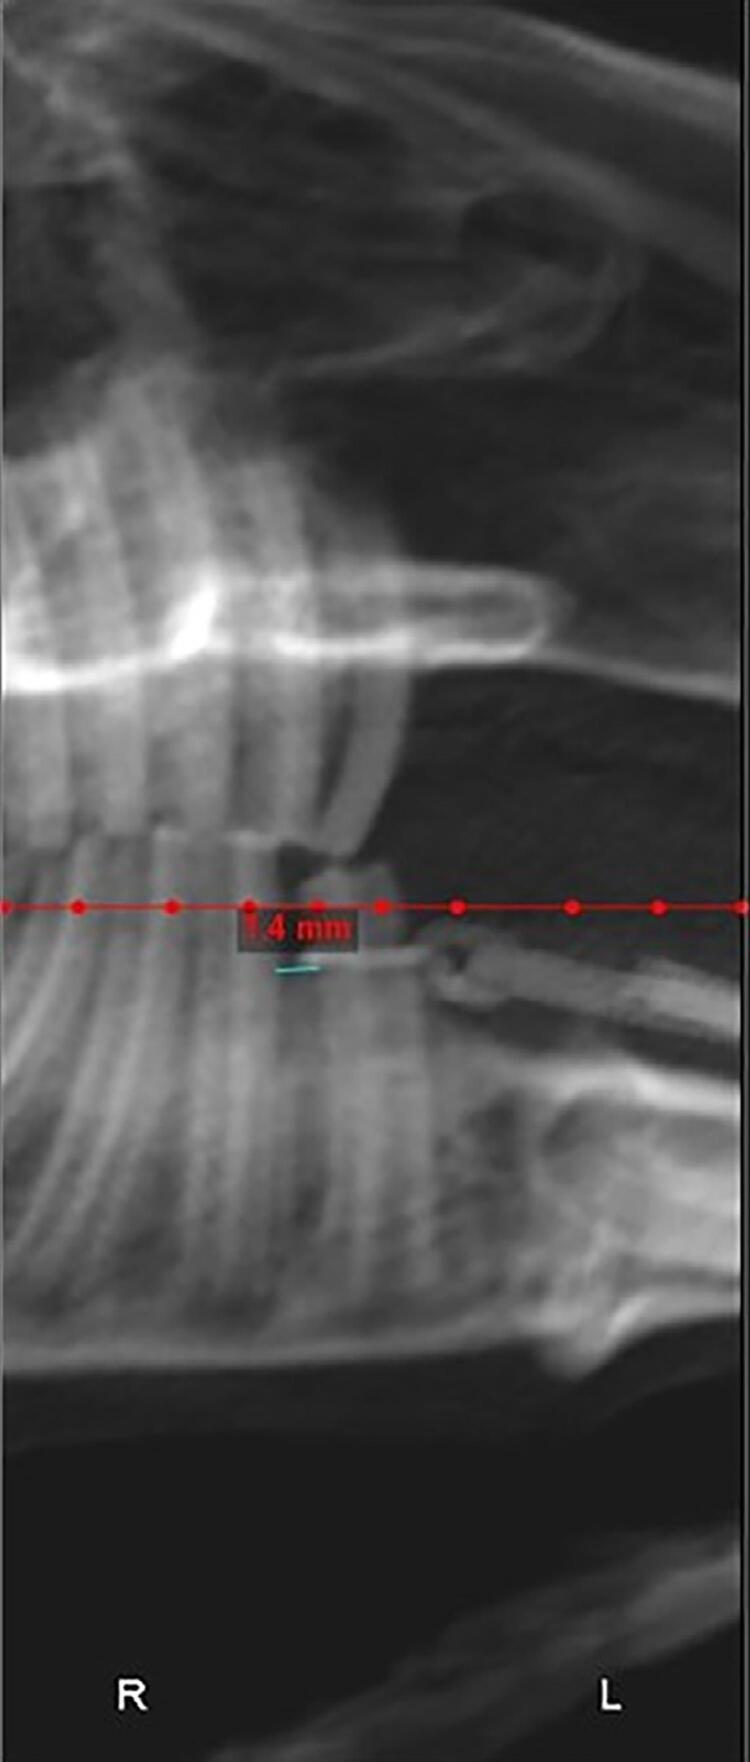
Computed tomography imaging for tooth movement distance measurement
